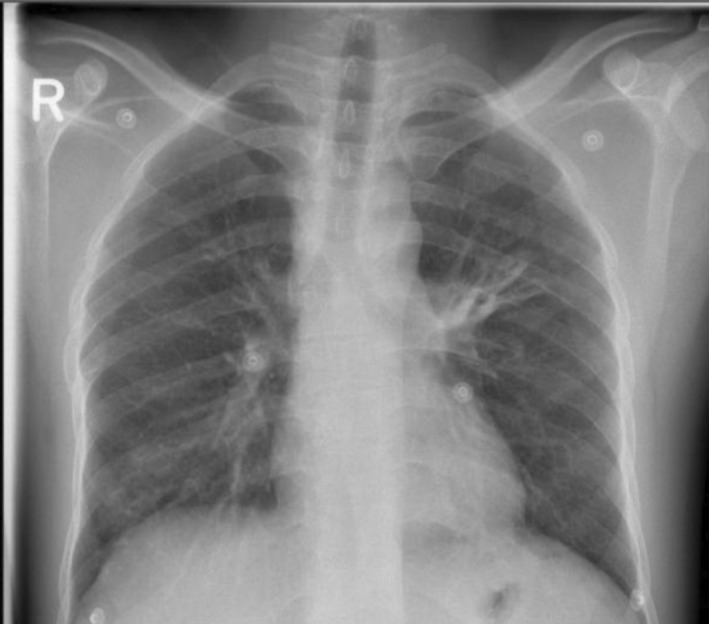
Postero‐anterior radiograph of the chest showing moderate regression in the previously seen left peri‐hilar patchy consolidation.
